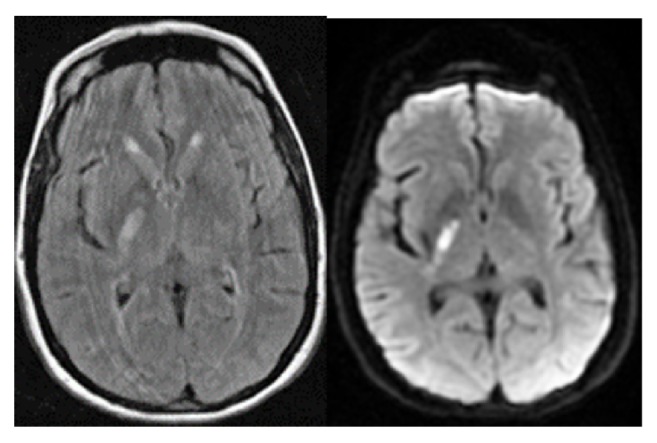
T2 FLAIR and DWI on initial presentation.
